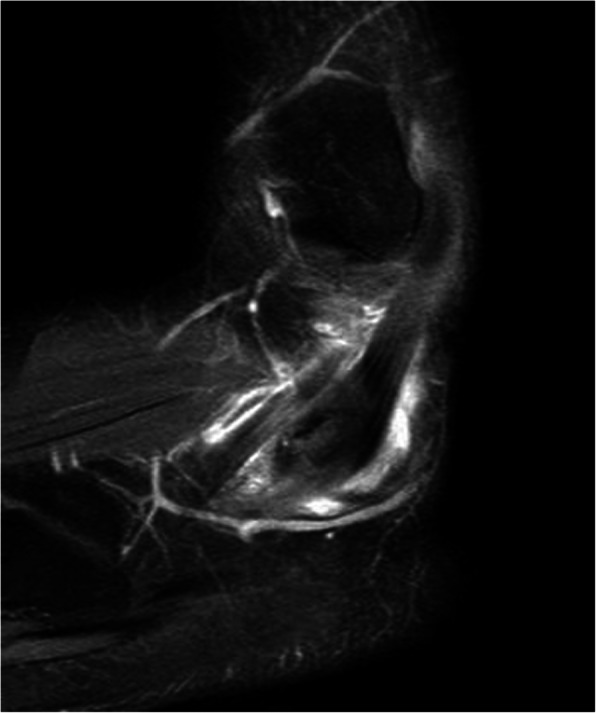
Sagittal MRI section of the enlarged and dislocated peroneus longus tendon over the peroneal tubercle
